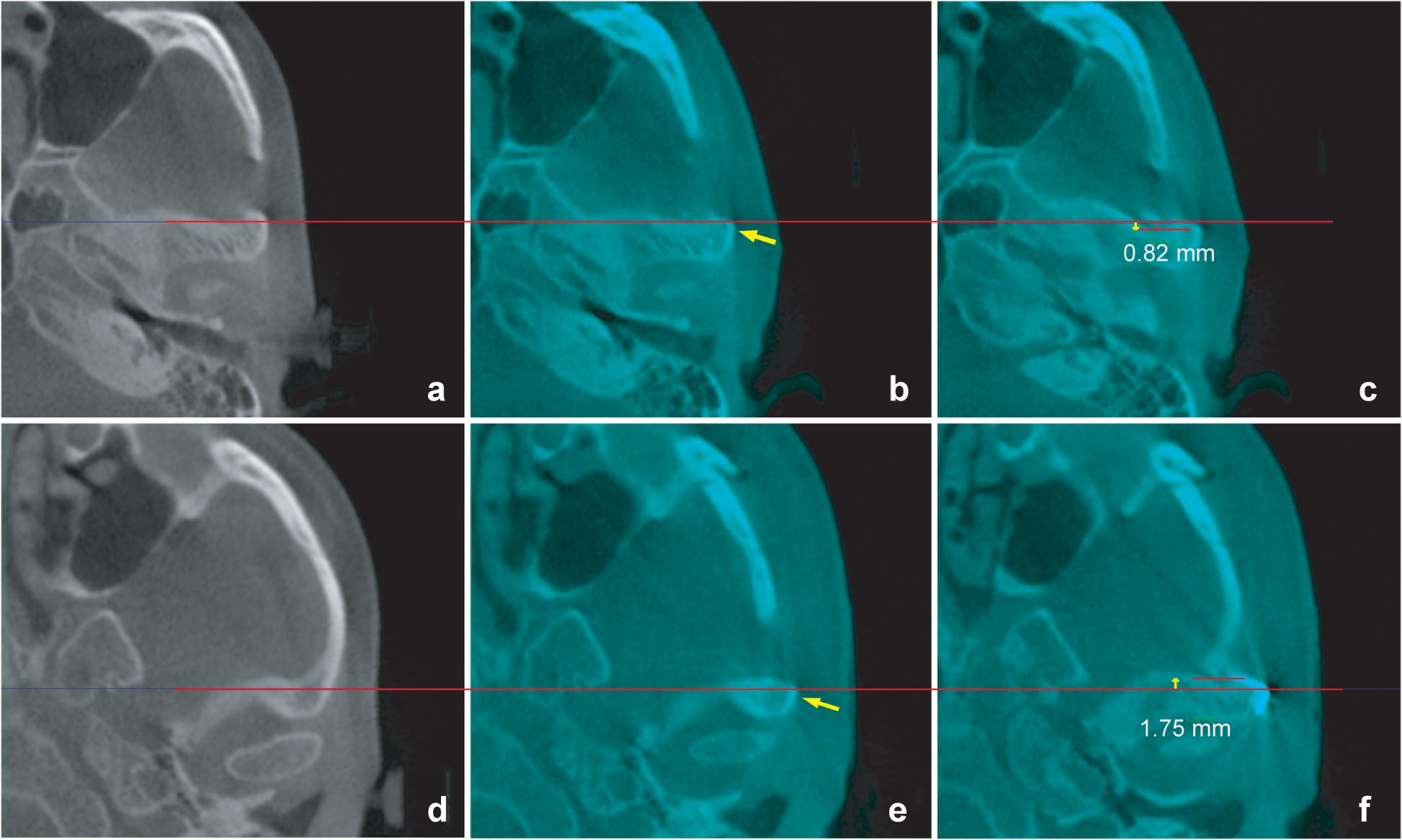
Measurement of the AP movement of the arch cutting edge on axial CT images. Comparison of the preoperative images (a and d) with the postoperative images (b and e) at the same level. The lateral cutting edge of the temporal bone is shown in the lowermost axial plane (yellow arrows in b and c). The cutting edge of the arch moved inward. When the cut edge moved posteriorly, a negative value was assigned; when it moved anteriorly, a positive value was assigned. The red line indicates the cutting edge baseline of the temporal bone. c and f show backward and forward movements of the arch, respectively
